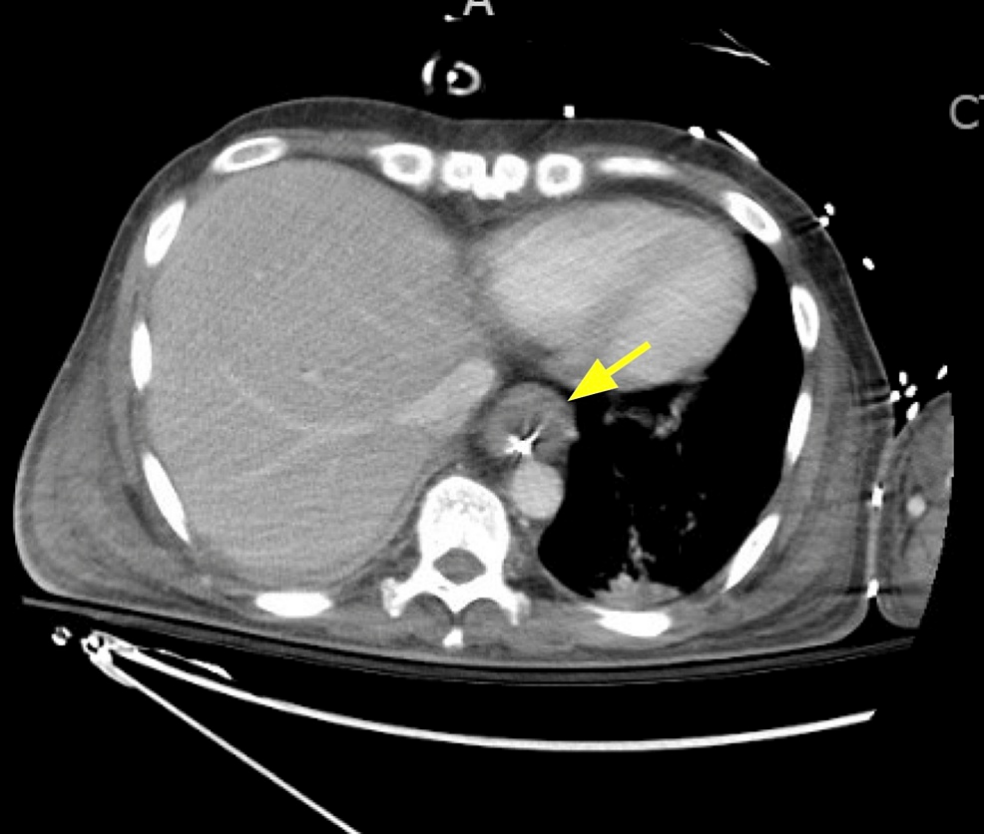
Computed Tomography of the Abdomen and Pelvis Demonstrated Lower Circumferential Esophageal Wall Thickening and Retroperitoneal Lymphadenopathy Arrow pointing to esophageal wall thickening
